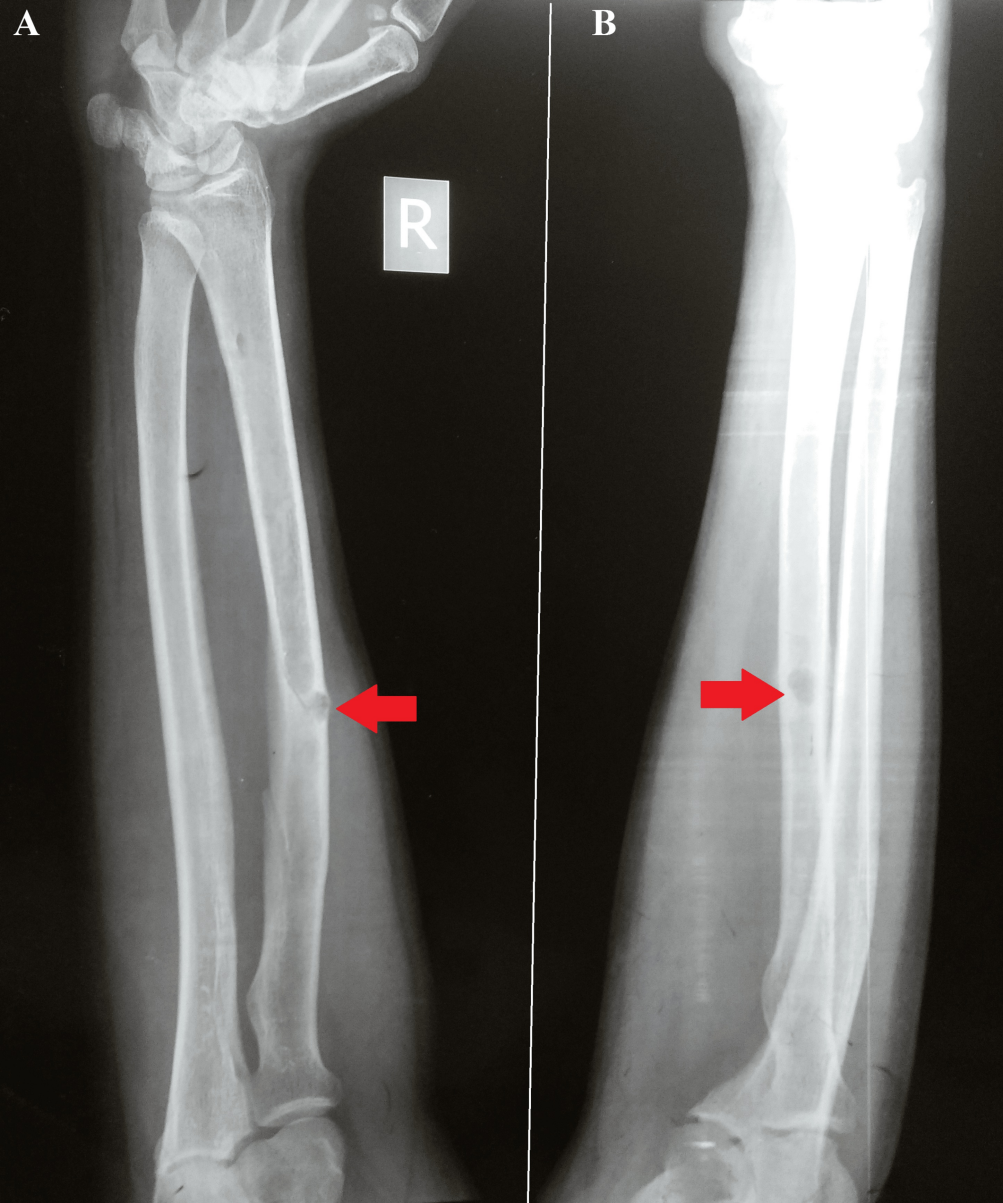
Plain radiograph of the left forearm and the wrist (A) Anteroposterior view. (B) Lateral view. Arrows indicate the lesions in the left radius.
